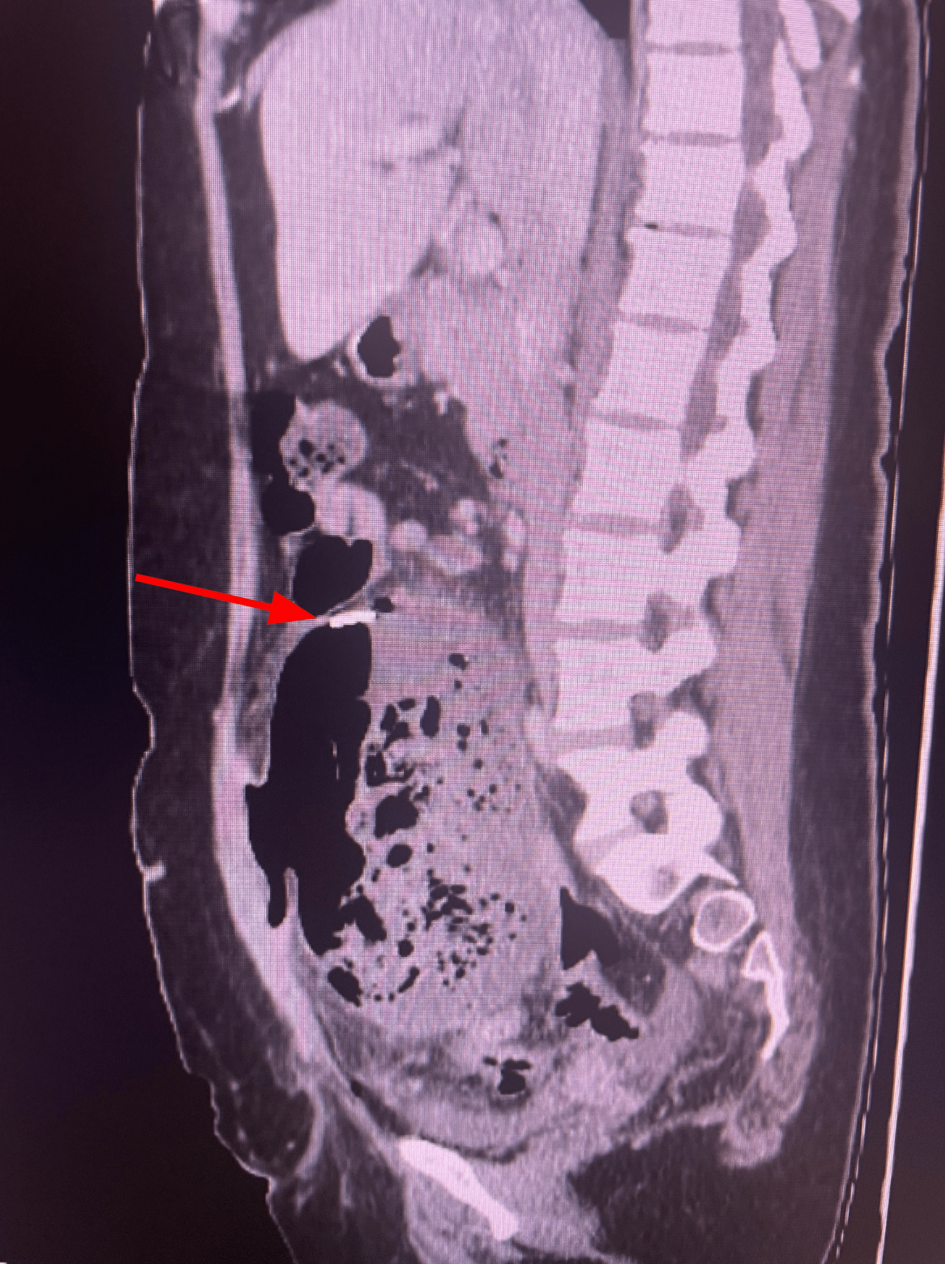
Sagittal CT shows a large intra-abdominal abscess in the lower abdomen and pelvis. A radiodense ribbon, indicated by the red arrow, is seen within the abscess cavity, consistent with a retained surgical towel
